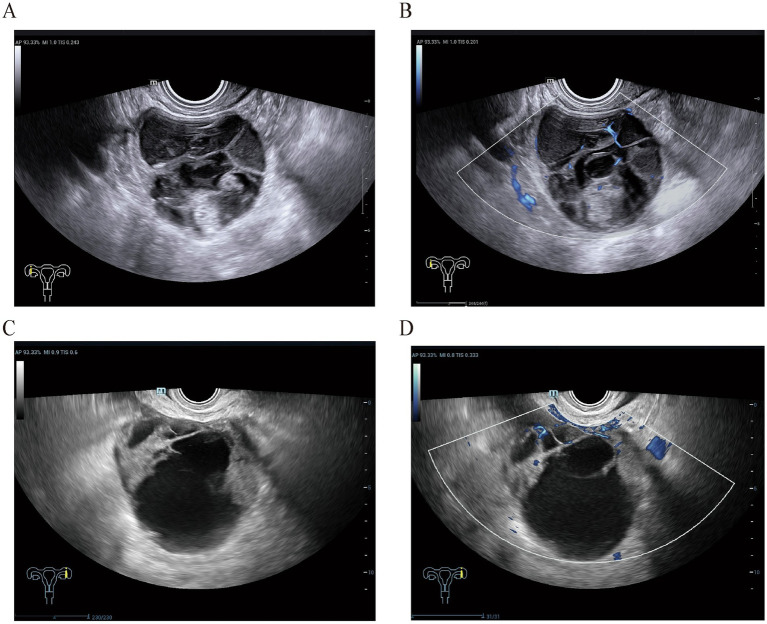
Ultrasonographic features of the ovary-adnexa. (A) Grayscale ultrasound findings: a cystic-solid mass, measuring approximately 8.4 × 8.1 cm, is observed superior to the left side of the uterus, with relatively clear boundaries. The cystic component demonstrates fair sound transmission. Internal septations with uneven thickness are seen. An irregular hyperechoic area protrudes from the cystic wall into the lumen, with the larger one measuring about 3.7 × 2.5 cm. (B) Color Doppler Flow Imaging (CDFI): a small amount of blood flow signal is detected on the septa, with a flow score of 2. Pathological diagnosis: left ovarian high-grade serous carcinoma. (C) Grayscale ultrasound findings: a well-defined cystic-solid mass, measuring approximately 6.5 × 5.5 cm, is seen in the right adnexal region, demonstrating poor sound transmission. The mass contains multiple locules with septations of variable thickness. A hyperechoic area is also identified within, with the larger one measuring about 1.9 × 1.2 cm. (D) Color Doppler Flow Imaging (CDFI): A small amount of blood flow signal is detected on the septa, with a flow score of 2. Pathological diagnosis: right ovarian multilocular mucinous cystadenoma.
